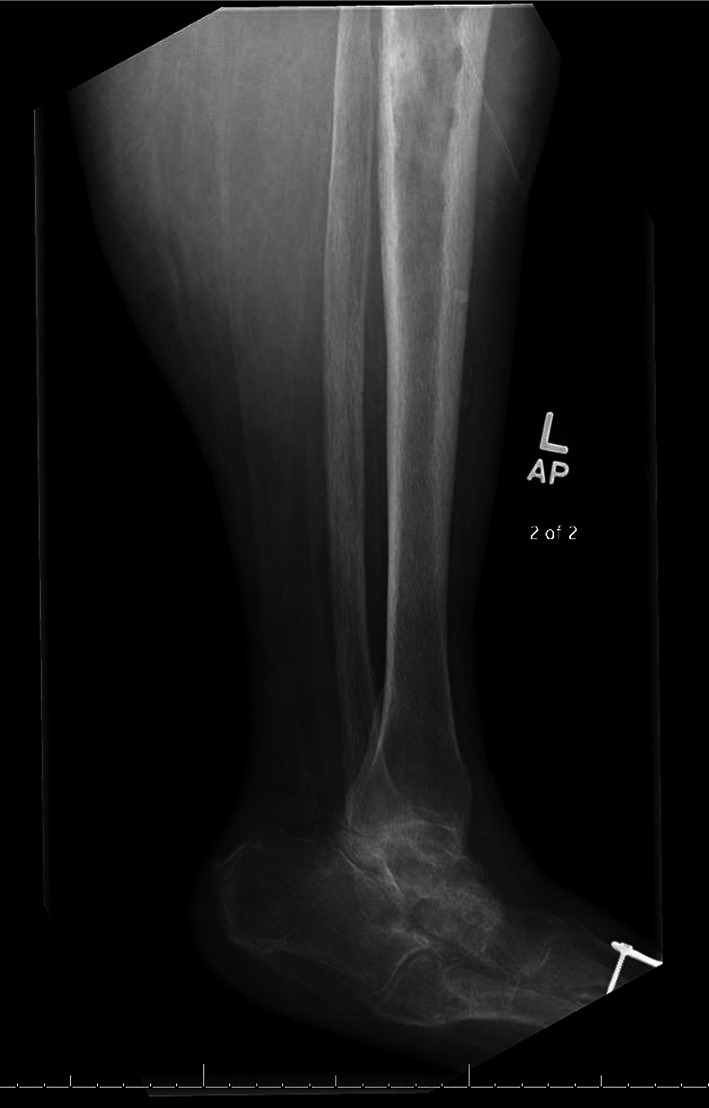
Plain radiograph of the patient’s left lower extremity demonstrating focal soft tissue swelling overlying the anterior aspect of the tibia
